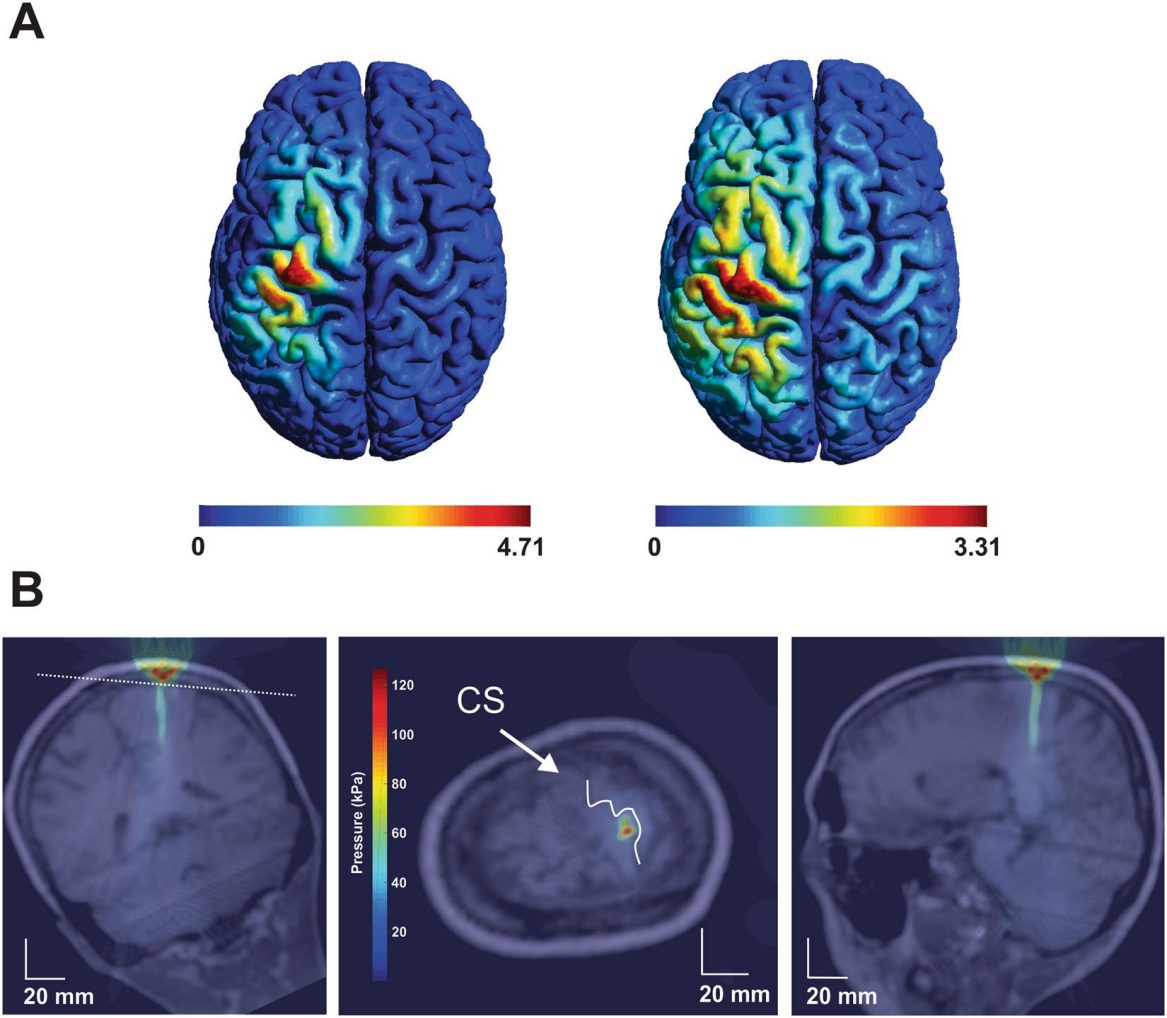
Modelled Ultrasound/TMS field and ultrasound field in the brain. (A) Modelled induced electric field in the human brain from the TMS coil without the ultrasound transducer (left) and with the ultrasound transducer (right). (B) Acoustic model of the ultrasound waveform positioned over primary motor cortex. CS = central sulcus. Note: Middle figure is transverse section taken through the plane (white dashed line) in the left coronal figure. kPa = kilopascals.
